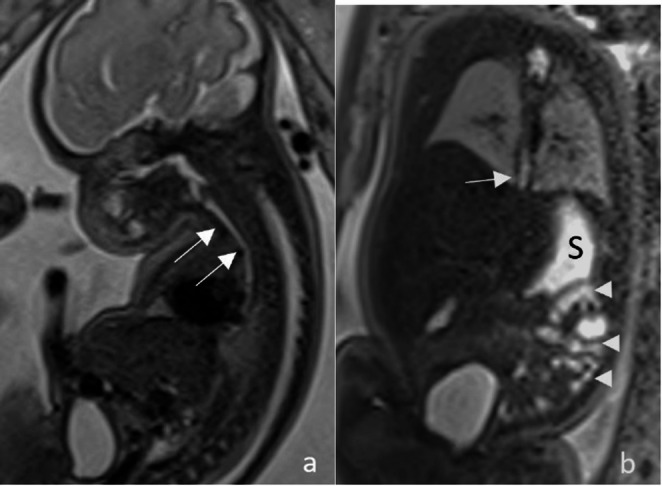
Normal morphology of the proximal digestive tract Sagittal (a) and coronal (b) T2-W MR images at 34 gestational weeks (GW) show the esophagus as a fluid-filled, regular thin tubular structure in the posterior mediastinum (arrows, a-b). Fluid filling the esophagus, stomach (S), and proximal small bowel has the same signal intensity as the amniotic fluid. Note the homogeneous diameter of the proximal bowel loops (head arrows, b)
